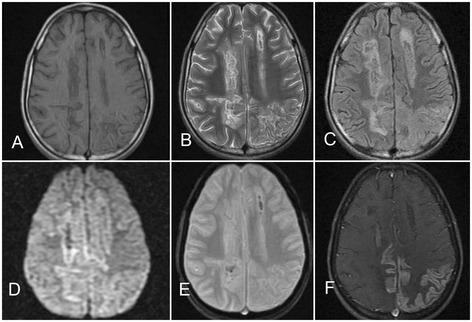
Axial sections of MRI of the brain depict curvilinear hyperintense cortical lesions in bilateral parietal and occipital areas on T1 W (a), T2 W (b) and T2 fluid attenuated inversion recovery (c) sequences. Mild gyral diffusion restriction is noted on diffusion weighted sequence (d) while blooming is seen on gradient echo sequence (e). Post-contrast T1 W sequence (f) depicts gyral enhancement
